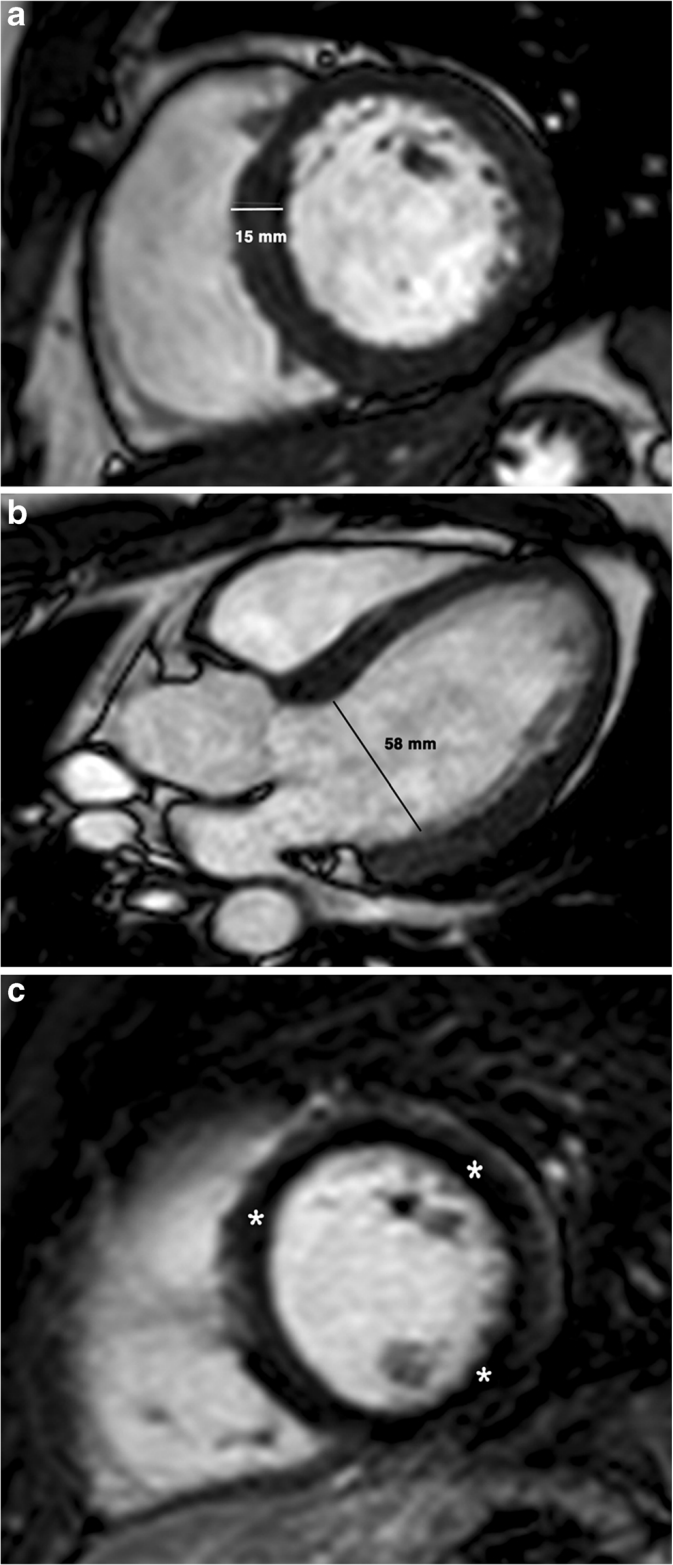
A 20-year-old competitive runner man with athlete’s heart. a Short-axis and (b) three-chamber steady-state free precession (SSFP) MR images at end-diastole. The left ventricle is enlarged and (end-diastolic diameter = 58 mm) and basal septal thickness is 15 mm. c Post-contrast T1-weighted inversion recovery gradient echo image shows normal myocardial signal intensity (*asterisks*)
